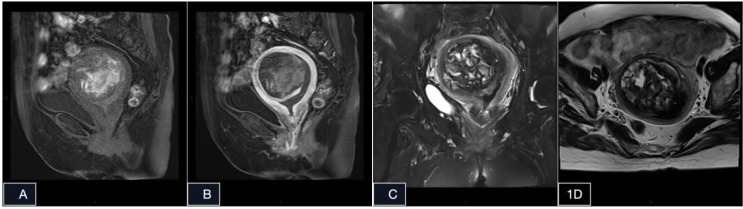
Precontrast T1-weighted (A),Postcontrast T1-weighted (B), Precontrast T1-weighted (C, D). Preoperative MRI showing a 6 cm × 5.5 cm × 5 cm tissue mass with heterogeneous signal which was identified in the anterior right wall of the uterus, predominantly exhibiting high signals on both T1 and T2-weighted imaging. Additionally, a few patchy delayed enhancements were observed during enhanced scanning.
